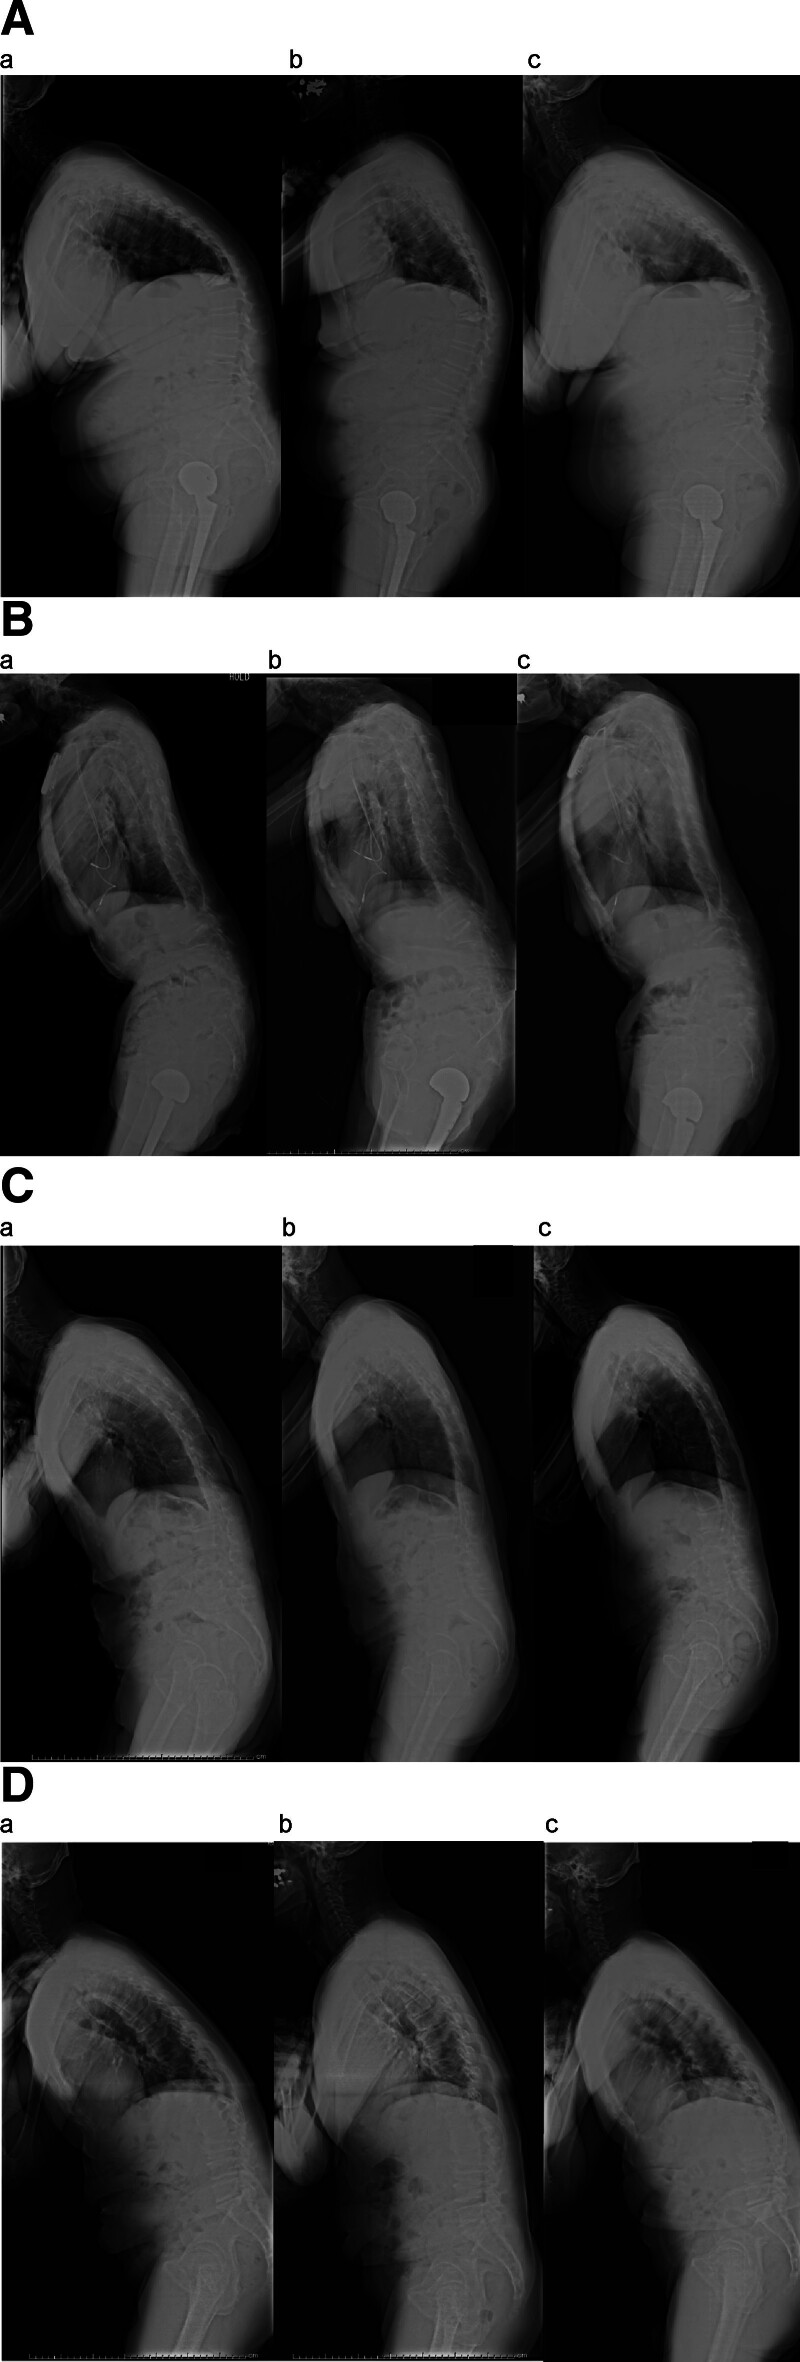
Time series of whole-body lateral radiographs to assess postural changes associated with treatment interventions. (A) Case 1: Radiographs of the spine were taken on the first day (a), 5 d later (b), and 1 mo later (c) after the injection. (B) Case 2: Radiographs of the spine were taken on the first day (a), 5 d later (b), and 1 mo later (c) after the injection. (C) Case 3: Radiographs of the spine were taken on the first day (a), 5 d later (b), and 1 mo later (c) after the injection. (D) Case 4: Radiographs of the spine were taken on the first day (a), 5 d later (b), and 1 mo later (c) after the injection.
